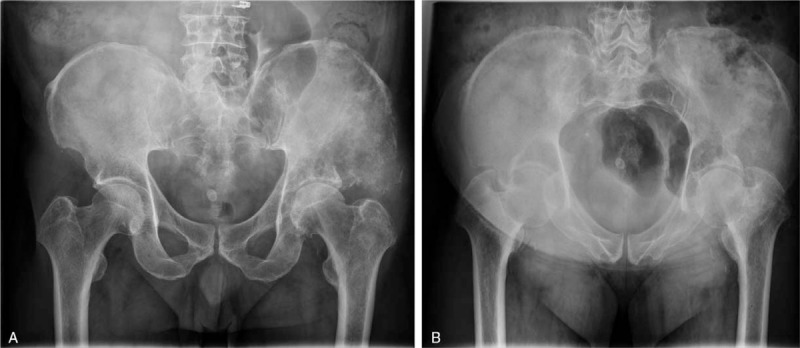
Preoperative pelvic radiographs. Anteroposterior view (A) and inlet view (B) show the osteolytic lesion in both the ilium and left acetabulum.
